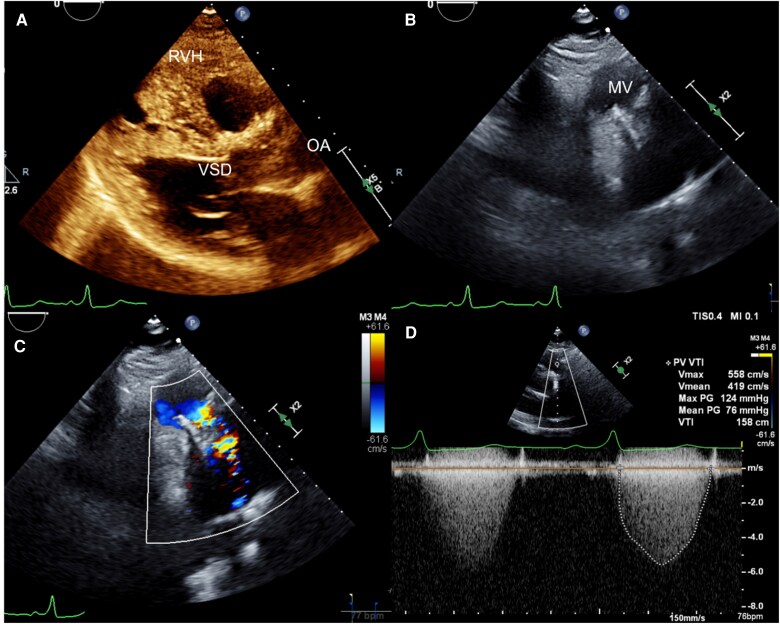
Transthoracic echocardiography at clinical presentation. (*A*) Parasternal long-axis view demonstrating findings consistent with tetralogy of Fallot including right ventricular hypertrophy, repaired ventricular septal defect, and overriding aorta. (*B*) Parasternal short-axis view of the pulmonic valve with prosthetic stent-mounted valve replacement. (*C*) Parasternal short-axis view of the pulmonic valve with colour Doppler demonstrating significant flow acceleration associated with prosthetic stenosis. (*D*) Continuous wave spectral Doppler across the prosthetic pulmonic valve in parasternal short axis demonstrating elevated peak velocity and mean gradient consistent with severe pulmonic stenosis.
